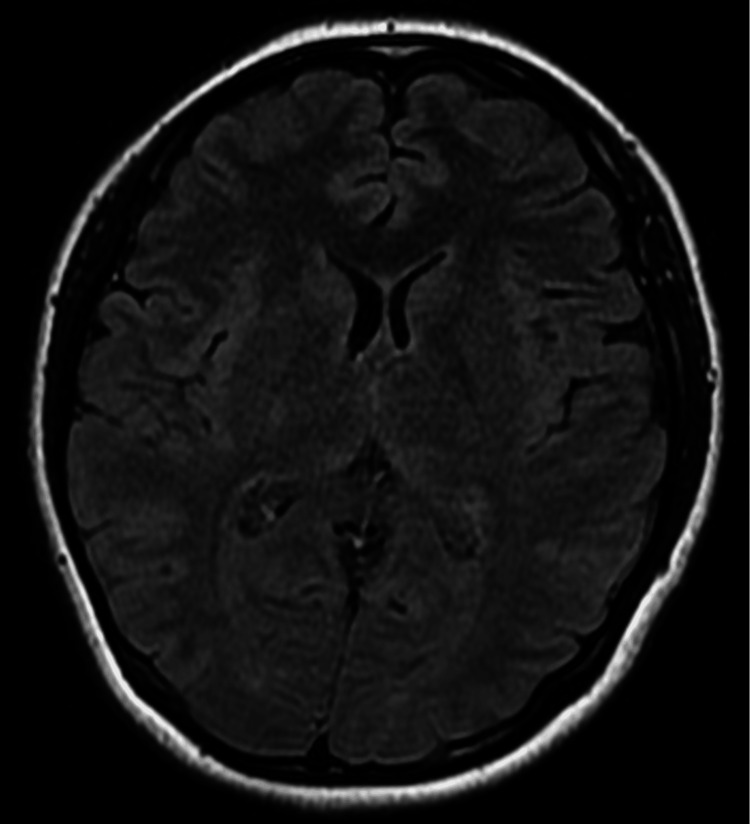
Brain MRI findings Brain MRI on T2-weighted FLAIR sequences showing no abnormalities, including in the basal ganglia MRI: magnetic resonance imaging; FLAIR: fluid-attenuated inversion recovery
